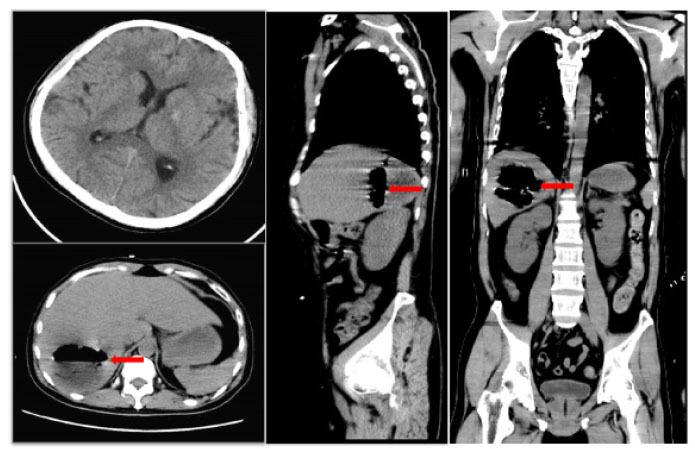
Initial brain CT scan shows no abnormalities, while sagittal and axial abdominal CT scans reveal a hepatic abscess with high gas and fluid levels in the right lobe. Red arrow: a large amount of gas is seen in the cavity of the liver abscess. CT: computed tomography.
